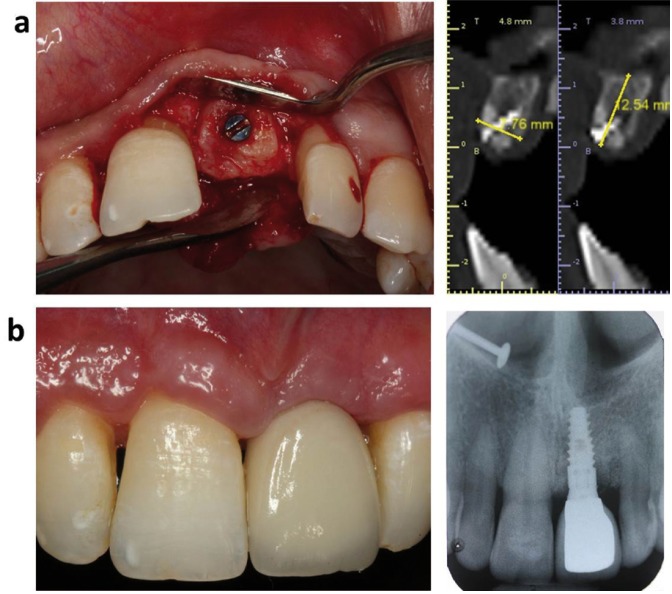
a) Post-augmentation clinical and radiographic view at 6 months recall. b) Clinical and radiographic view after Implant Restoration.
